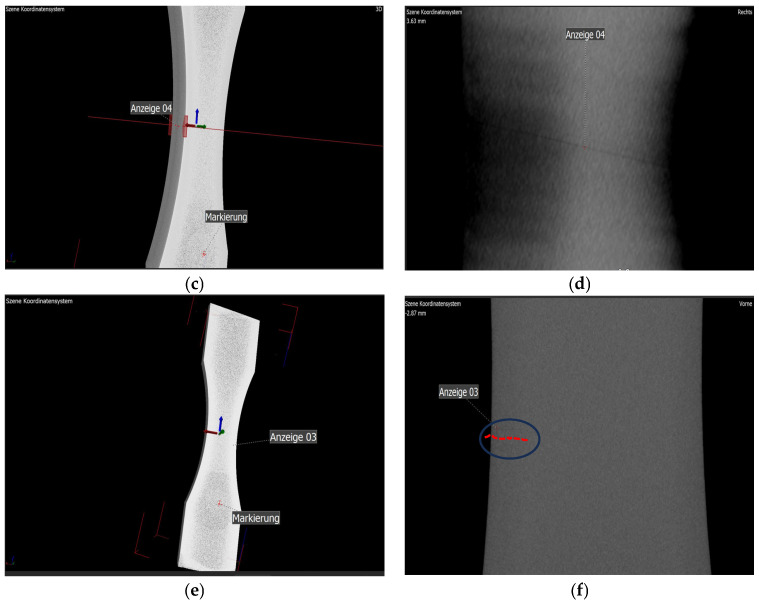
Non-destructive analysis applied to hardened and tempered sample after fatigue testing: (a,b) liquid penetrant inspection, (c) overview of CT examination: detail volume image—radius area, (d) microcrack measurement in radius area, (e) overview of CT examination: detail volume image—frontal surface of sample, and (f) microcrack measurement in the frontal surface area. The area highlighted in red represents the microcrack length, and the blue circle marks the region where the microcrack is present.
